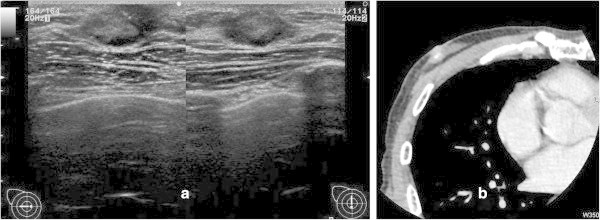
Ultrasonography (a) and computed tomography (b) showed a tumor behind the nipple with neither node enlargement nor distant metastasis.
